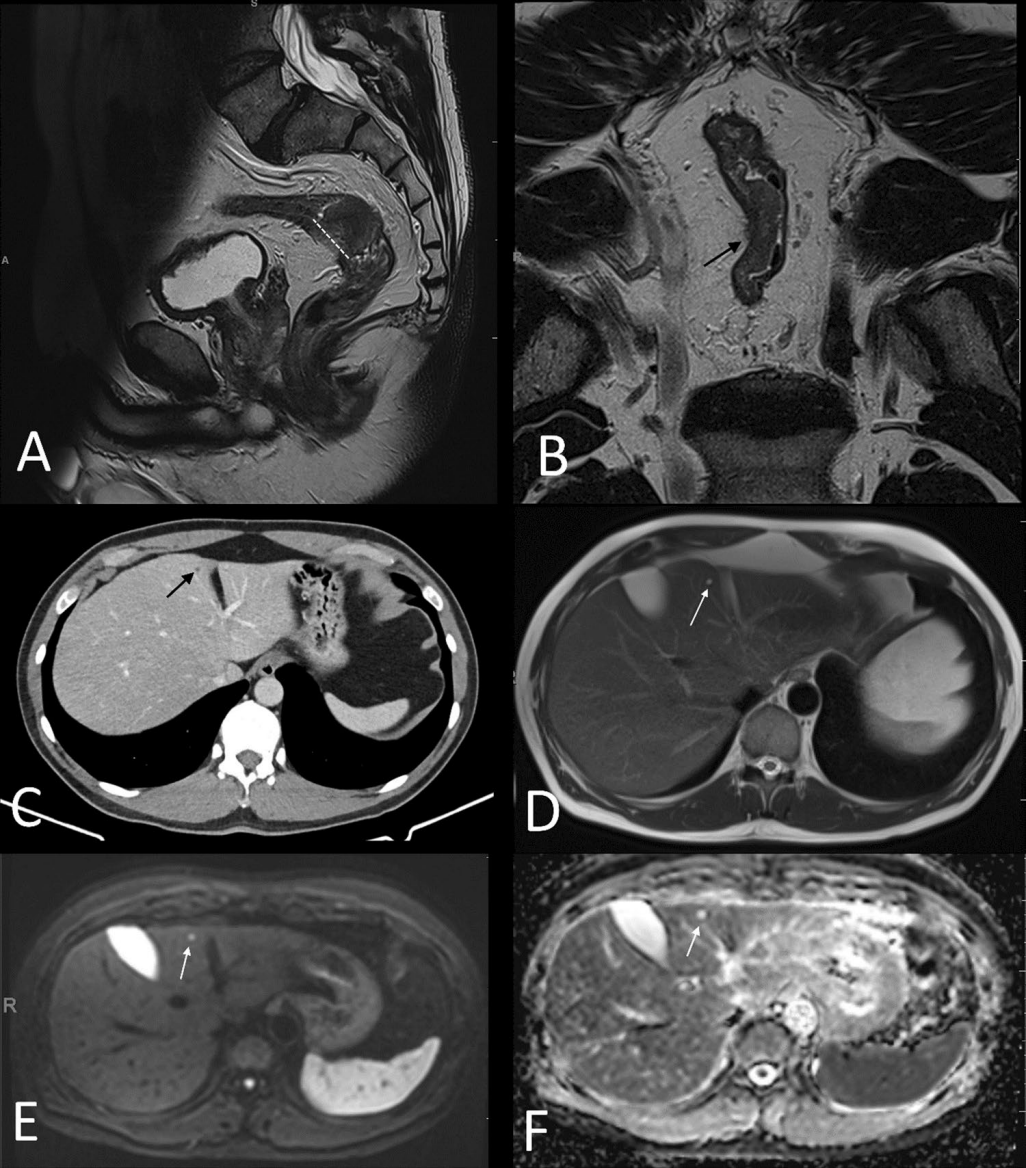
A and B sagittal and high-resolution coronal oblique images of a low-risk T3b N0 EMVI-negative CRM-negative PSW-negative mid-rectal tumor; dashed white line delineated the maximal length of the tumor, solid black arrow shows the 3 mm of extramural spread consistent with T3b disease; C contrast-enhanced CT that showed multiple too small to characterize hypodense liver lesions; D T2-weighted MRI of the liver that showed multiple small high T2 liver lesions, more numerous than shown on the CT; E and F B400 series of the diffusion weighted sequence (E) and the corresponding ADC map (F) showing the multiple liver lesions to be high signal on both sequences consistent with T2 shine through and not true restricted diffusion, confirming the lesions to be small, benign hepatic cysts; there were no suspicious lesions identified; in this case abbreviated liver MRI confirmed the multiple too small to characterize lesions identified on CT to be benign
